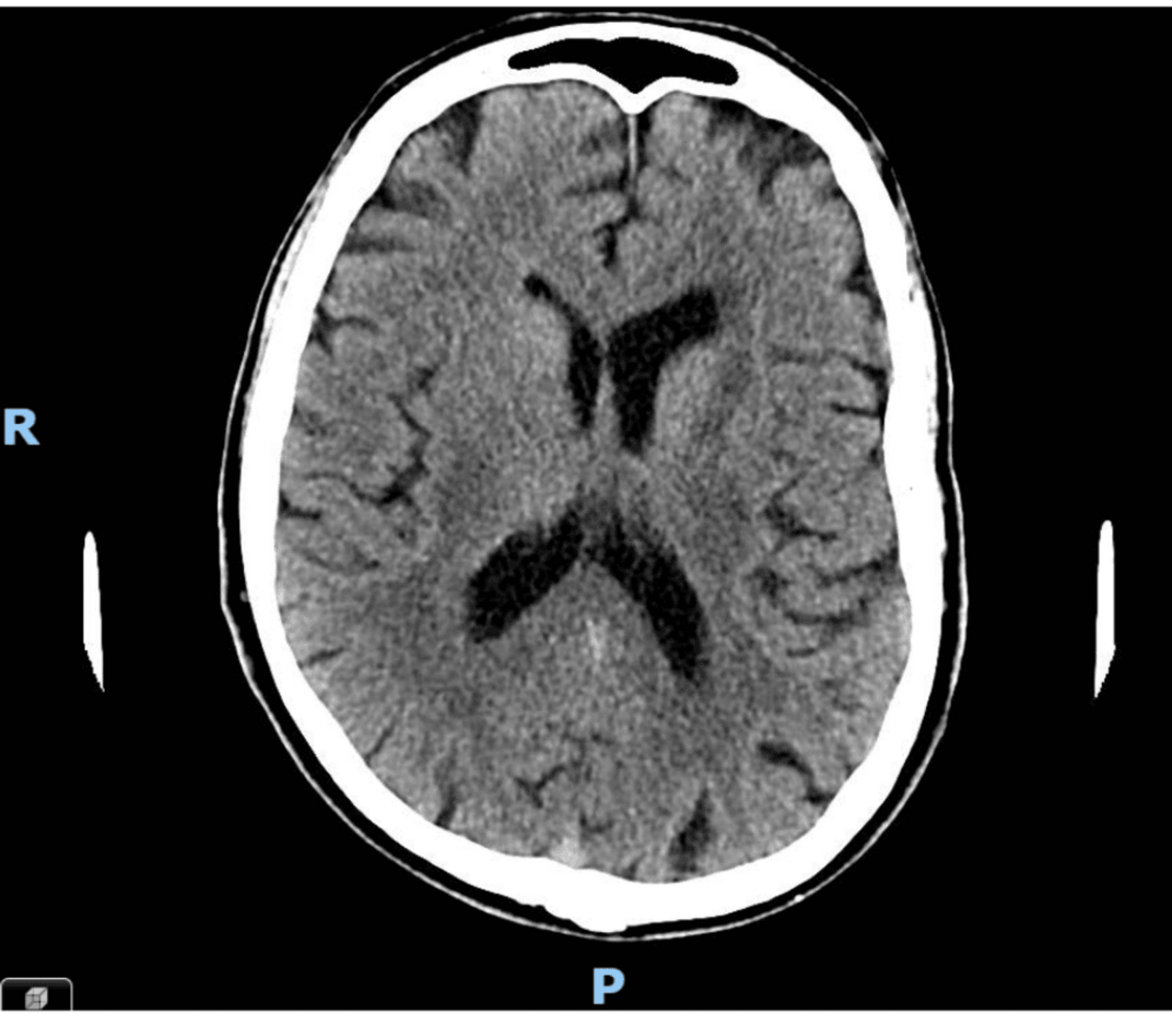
Non-contrast CT head No evidence of intracranial hemorrhage, acute infarction, or obvious space-occupying lesion was noted at the time of reporting. Ventricles and CSF cisterns were initially reported as normal. However, retrospective review in light of subsequent MRI findings revealed subtle asymmetry between the lateral ventricles, suggestive of early right-sided mass effect.
